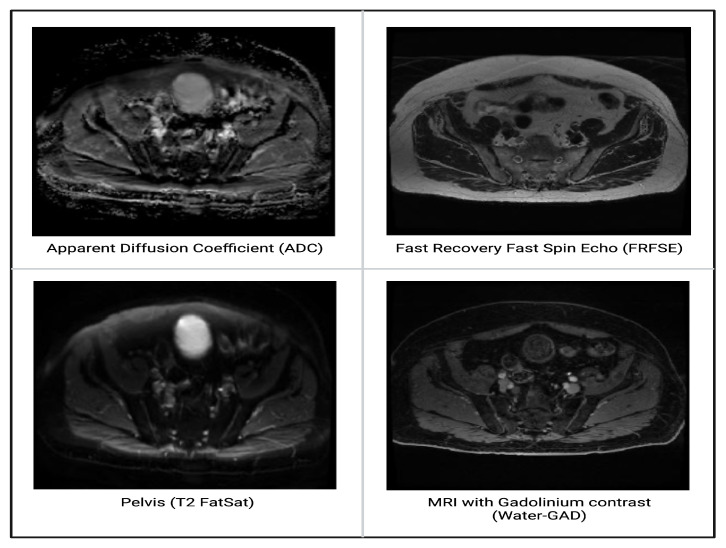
A prostate MRI from the same patient; four MRI sequences are shown.
